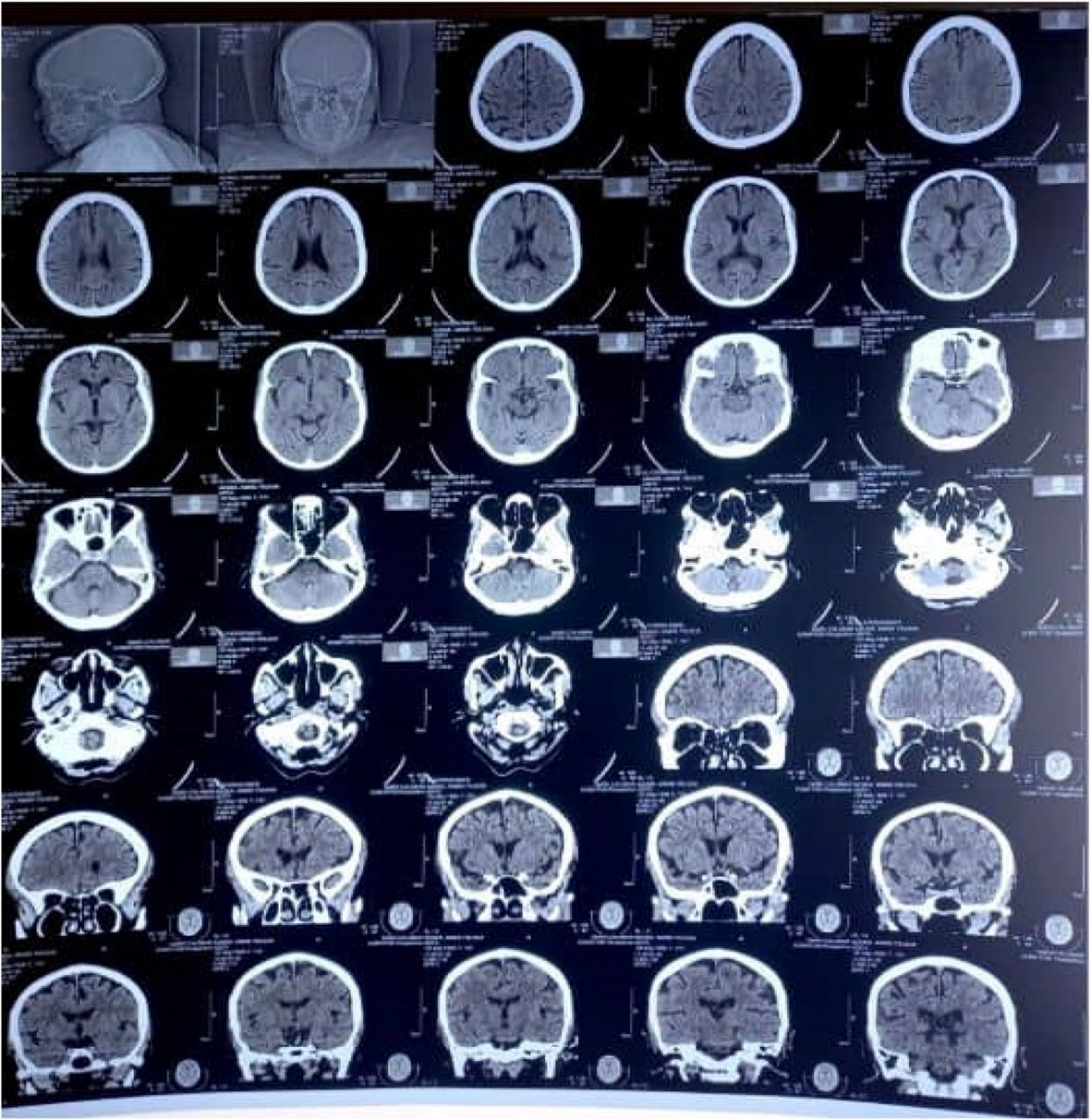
Cranial CT scan showing mild age-related cerebral atrophy, no focal intracerebral, or intracerebellar mass lesion seen
